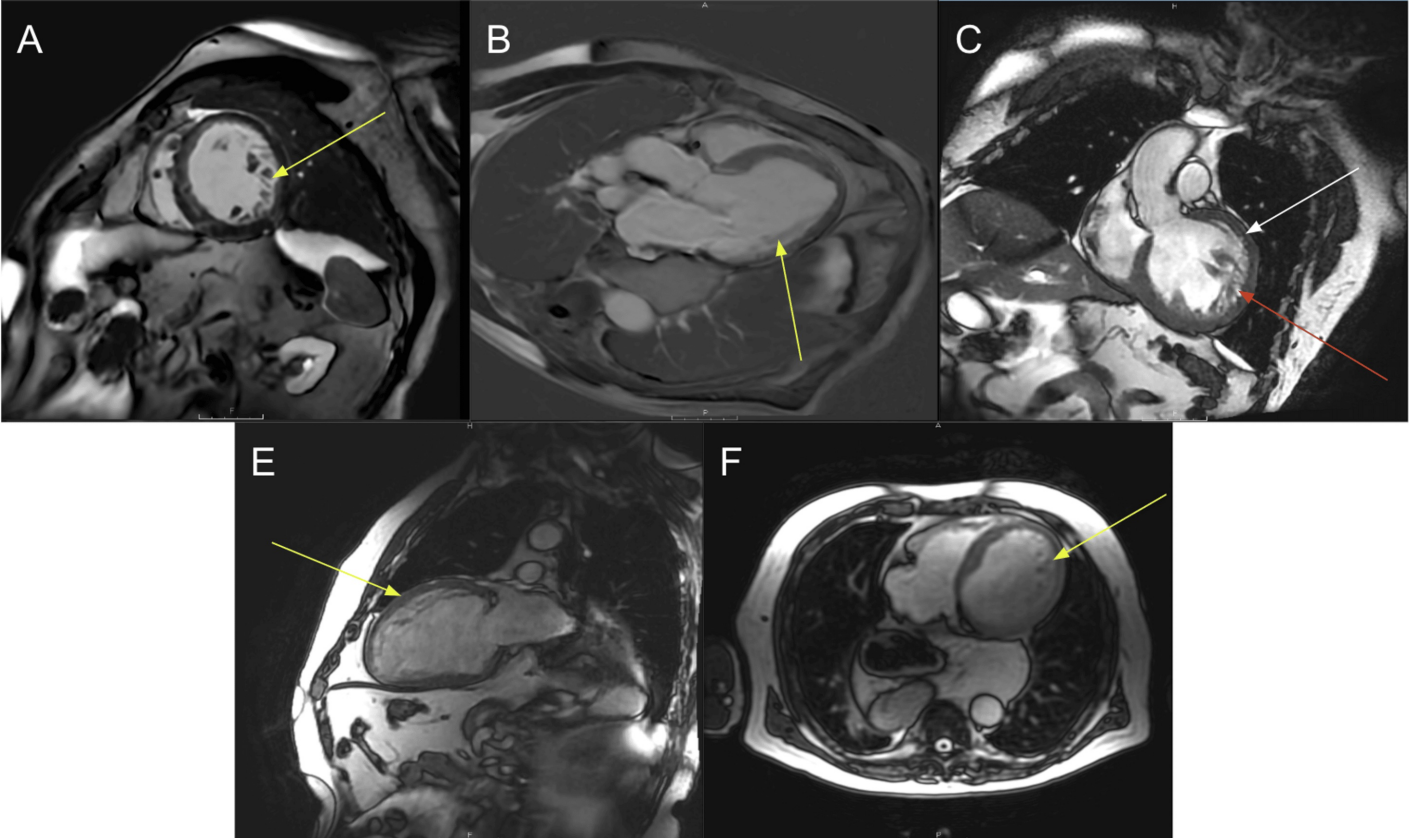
Cardiac MRI (A) Sagittal. (B) Short-axis apical. (C) Three-chamber vertical long axis. (D) Coronal. (E) Short-axis mid-diastole. (F) Axial. Cardiac MRI images reveal an increased trabeculated-to-non-compact myocardium in the left ventricle (yellow arrows). The ratio of non-compacted (white arrow) to compacted myocardium (red arrow) is 2.5.
